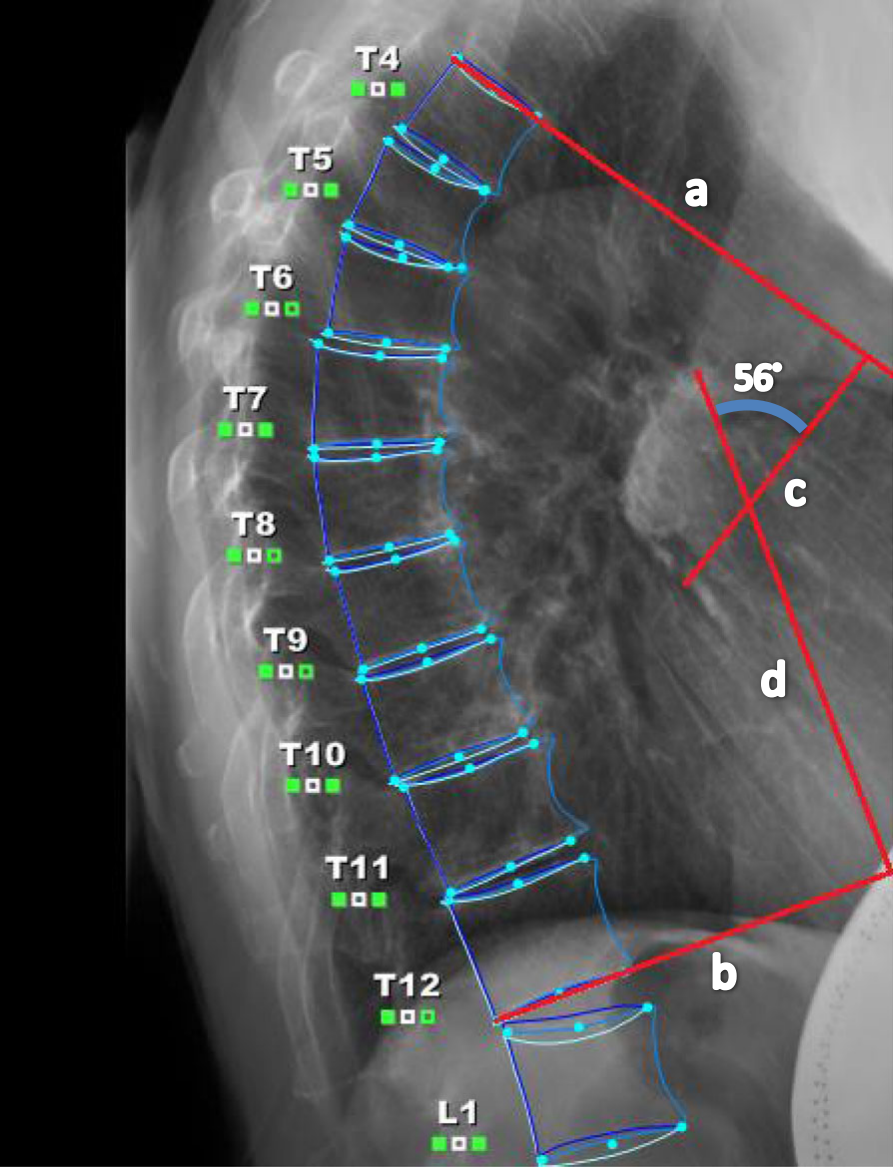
Cobb angle of kyphosis (56 degrees) measured from standing lateral radiograph Line a is drawn from the superior endplate of T4; line b is drawn from the inferior endplate of T12; lines c and d are perpendicular lines drawn from lines a and b. Cobb angle of kyphosis (56 degrees) is where lines c and d intersect
